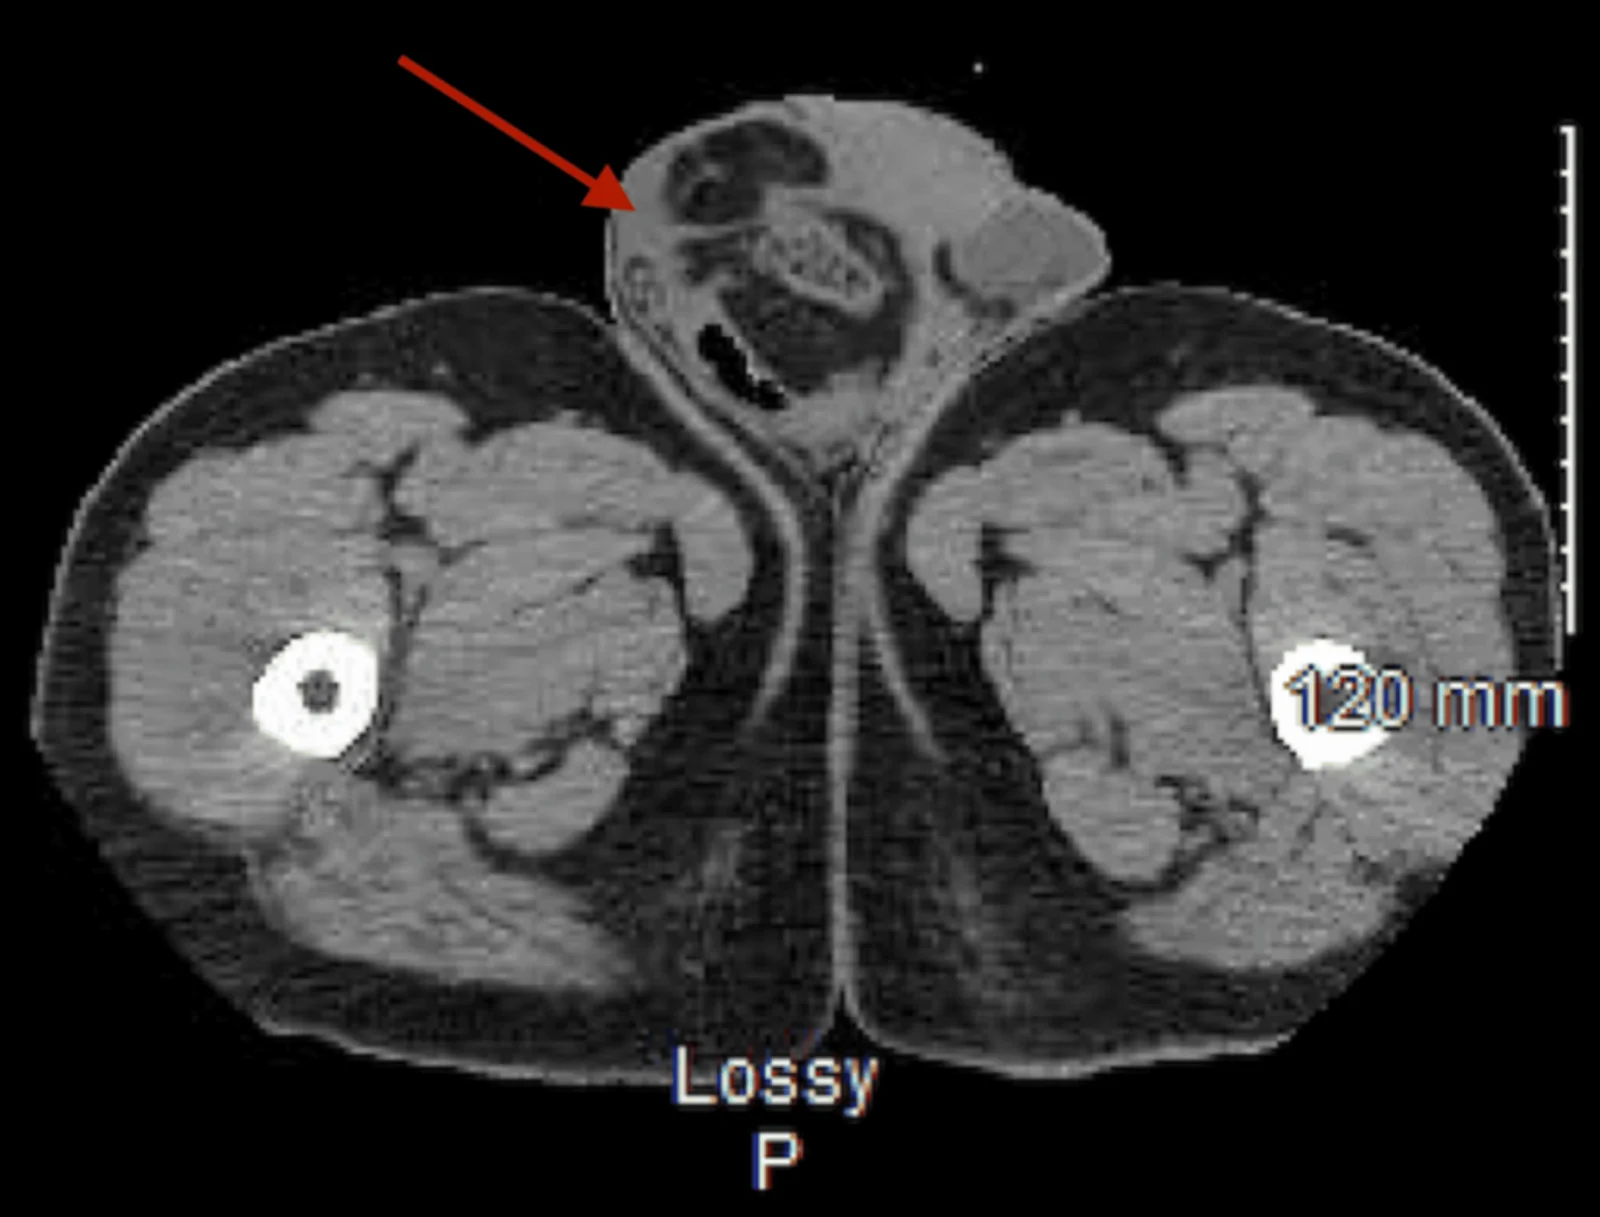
Apparent extension along the right inguinal region. Preoperative CT suggests extension toward the right hemiscrotum along the inguinal region (red arrow). Operative exploration subsequently demonstrated that the ventral component traveled through a subcutaneous plane superficial to the inguinal canal.
